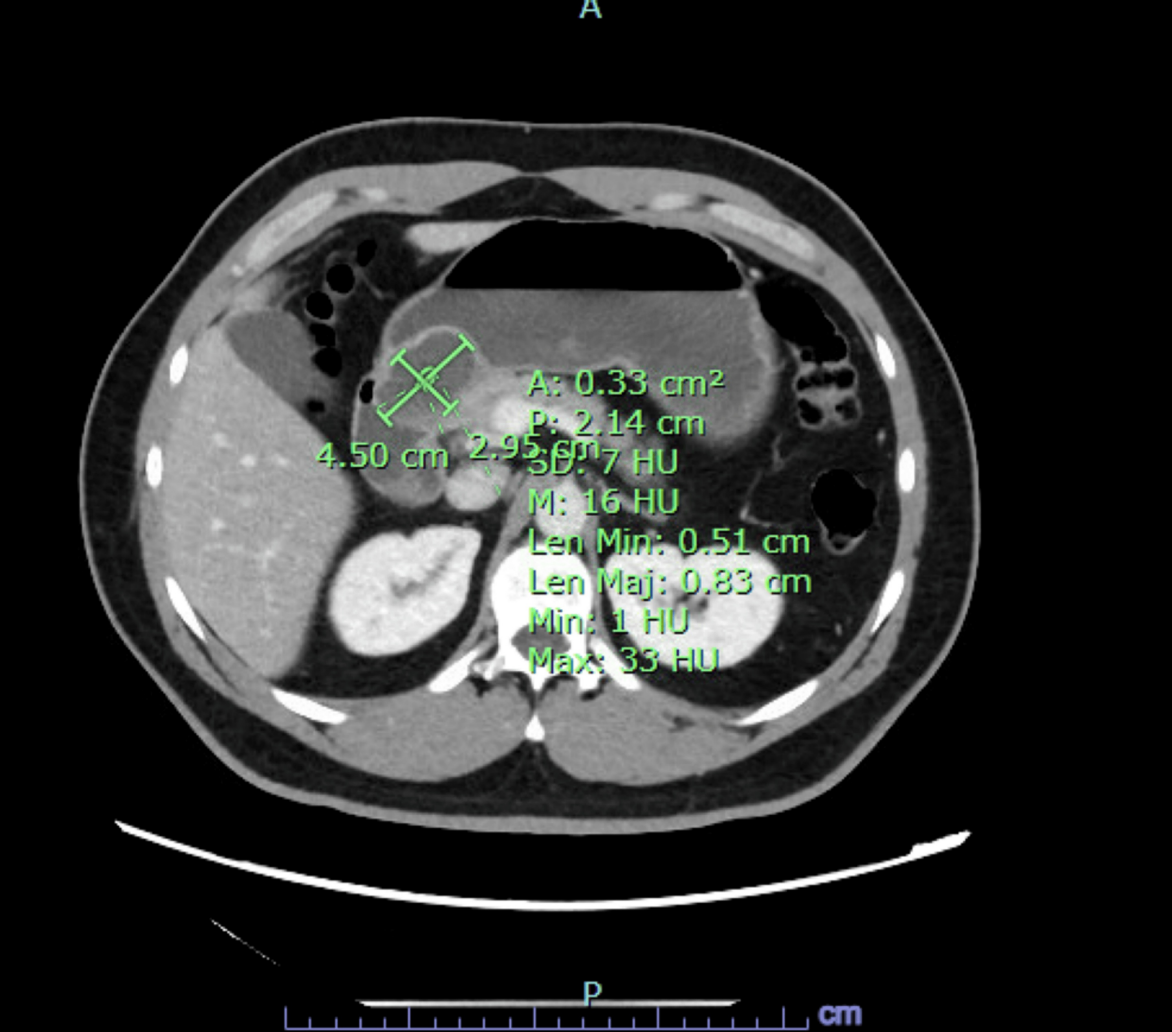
Initial CT identification of gastric outlet obstruction. CT of the abdomen obtained upon initial presentation, revealing a 4.5 × 3.0 × 2.9 cm cystic mass in the distal pylorus.
